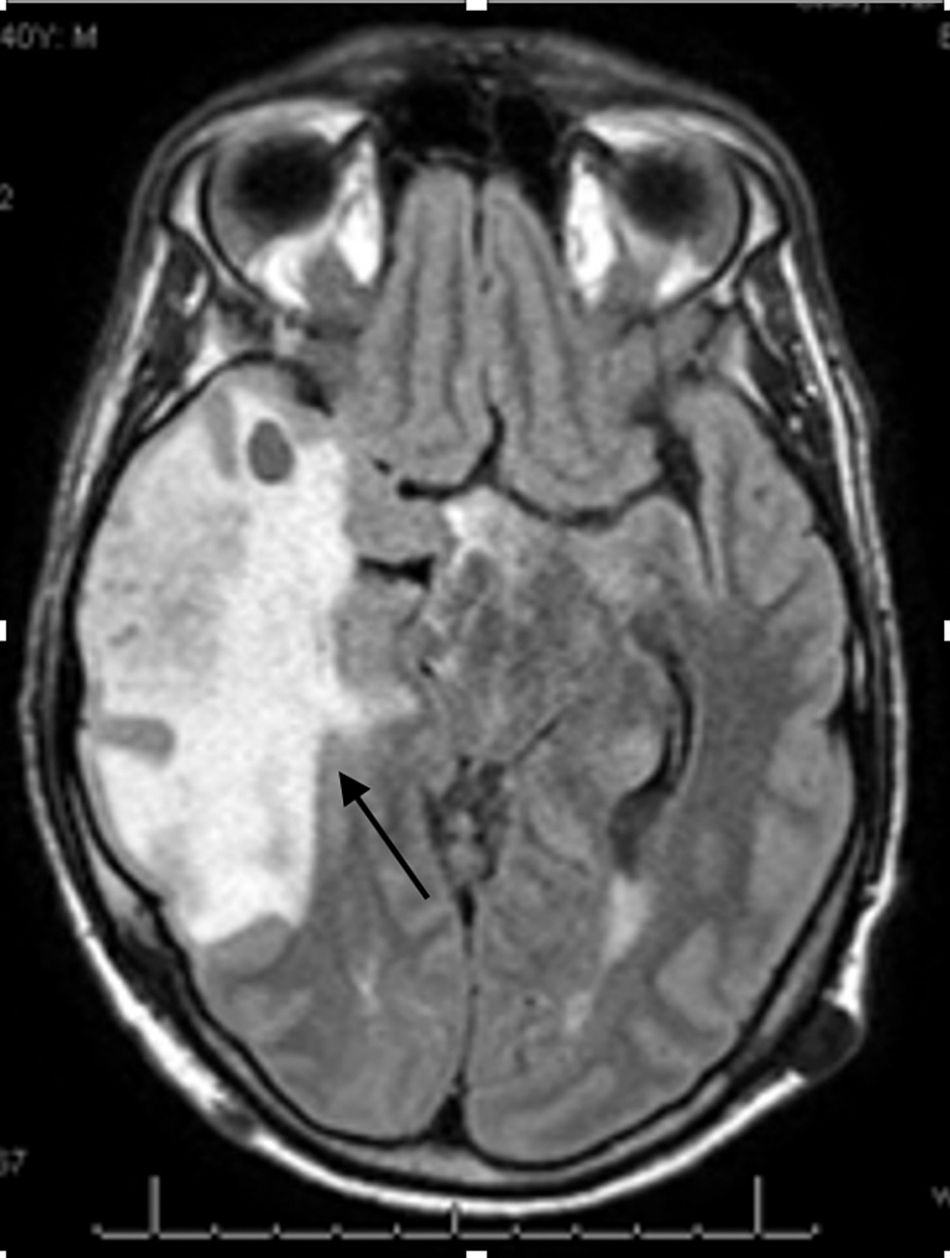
Progression of radiation necrosis Interval increase in size of the enhancing lesions within the right temporal lobe with related increase in surrounding T2/FLAIR hyperintense signal and increase in mass effect upon the right lateral ventricle and midline shift to the left.
